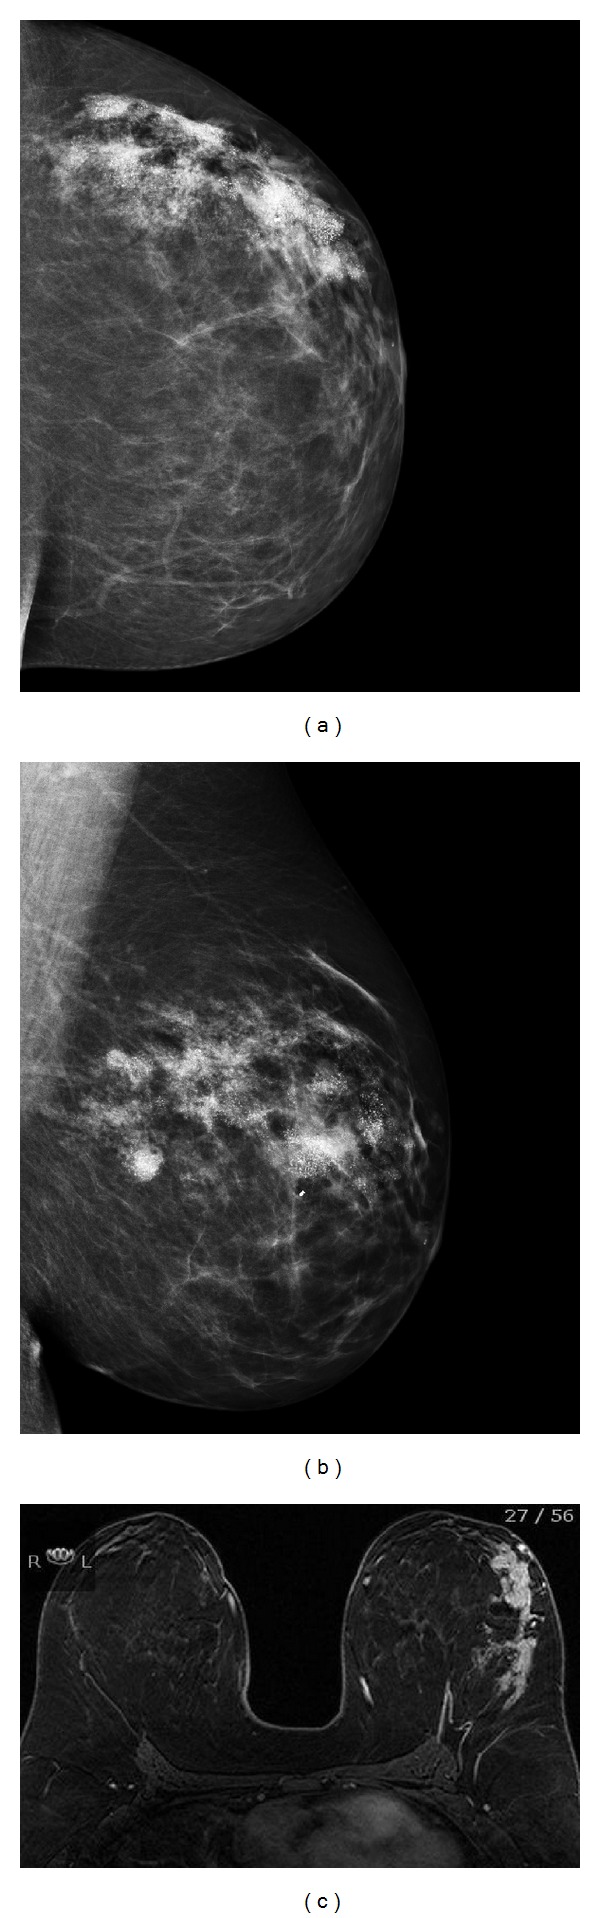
Representative imaging of patient in Case 1. Left mammogram showing clustered calcification corresponding to multiple sites of disease in craniocaudal (a) and mediolateral-oblique (b) projections. MRI showing extensive nonmass enhancement in the lateral hemisphere of the left breast in segmental distribution (c).
